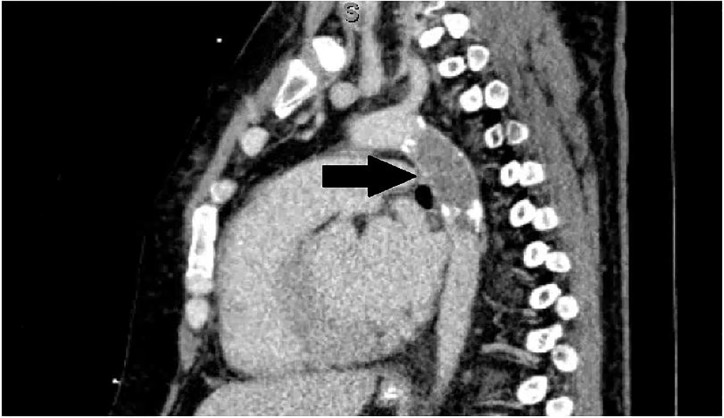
Computed tomographic angiography of the chest in sagittal view demonstrates complete occlusion of the proximal descending thoracic aortic stent (arrow).
